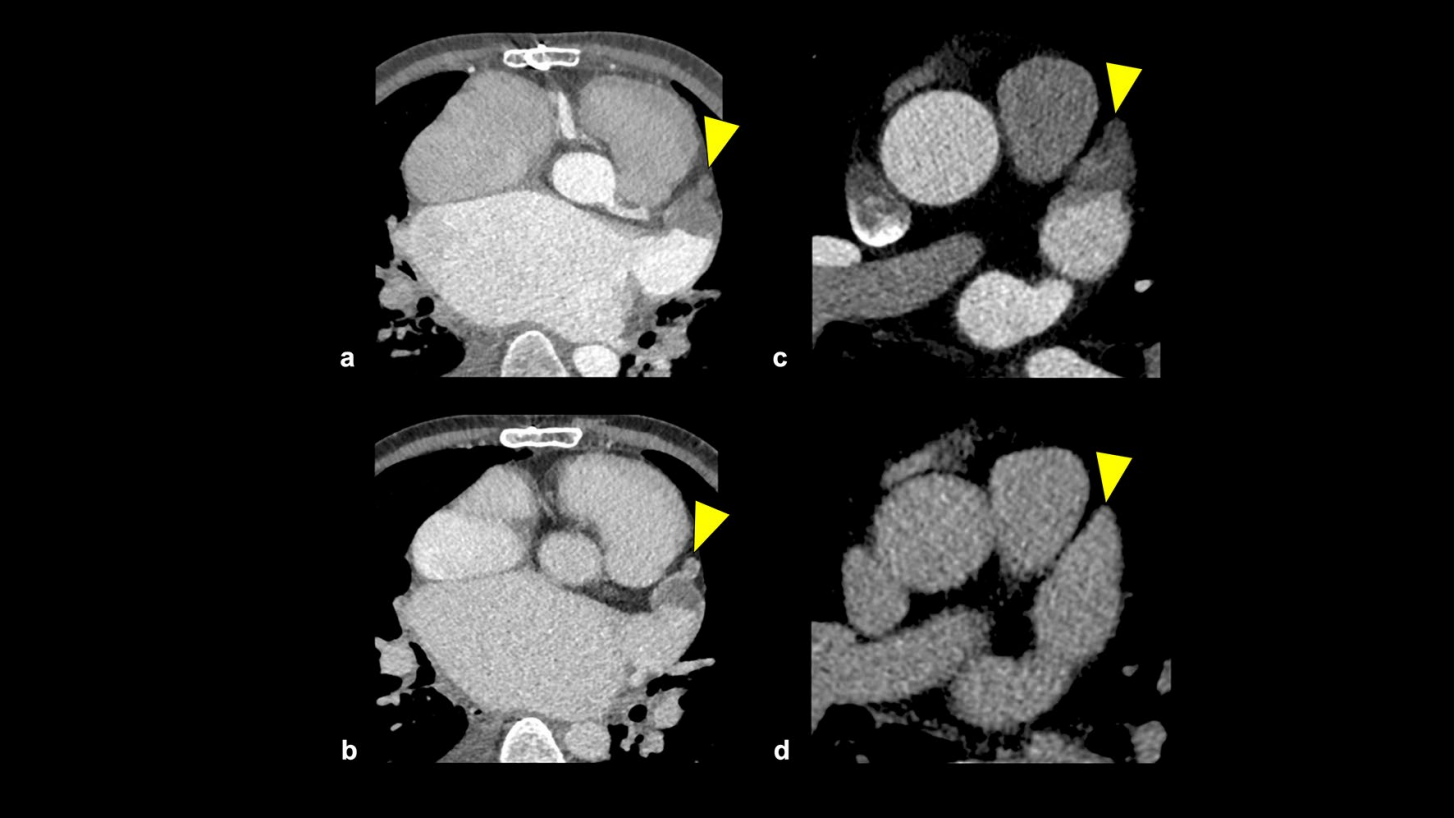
Left atrial appendage thrombus and slow-flow state. Both early-phase and delayed-phase CT images show a contrast defect in the LAA (a, b: arrowhead), and this case is suspected of the LAA thrombus. Early-phase CT image shows a contrast defect in the LAA (c: arrowhead), but the delayed-phase CT image shows no contrast defect in the LAA (d: arrowhead). This case is suspected of a slow-flow state. *LAA* left atrial appendage
